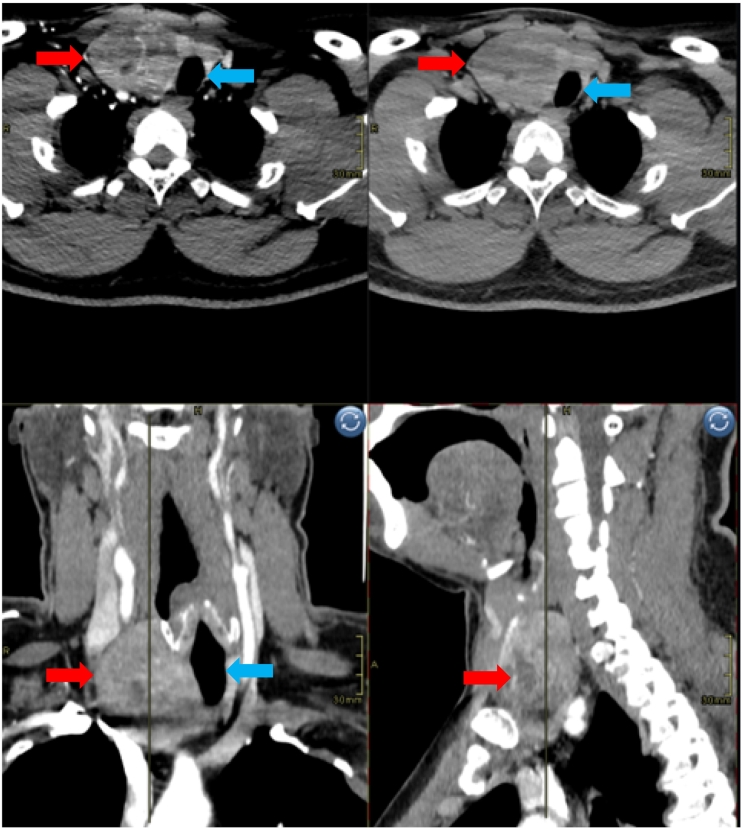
CT scan of neck (axial, coronal and saggital view) – Reformatted images of CT neck pre and post contrast showing a heterogneously enhancing solid mass involving the right thyroid lobe (red arrow) compressing and displacing trachea to left (blue arrow).
